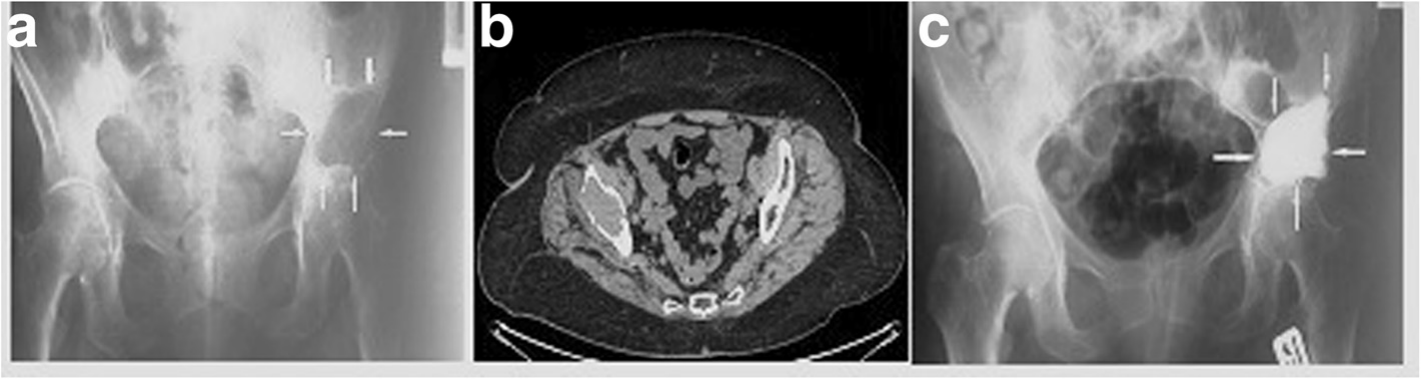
Anteroposterior radiogram (a) and transverse CT scan (b) in a kidney cancer patient with lytic bone loss in the right periacetabular area. Follow-up radiogram after bone cement (PMMA) augmentation (c)
